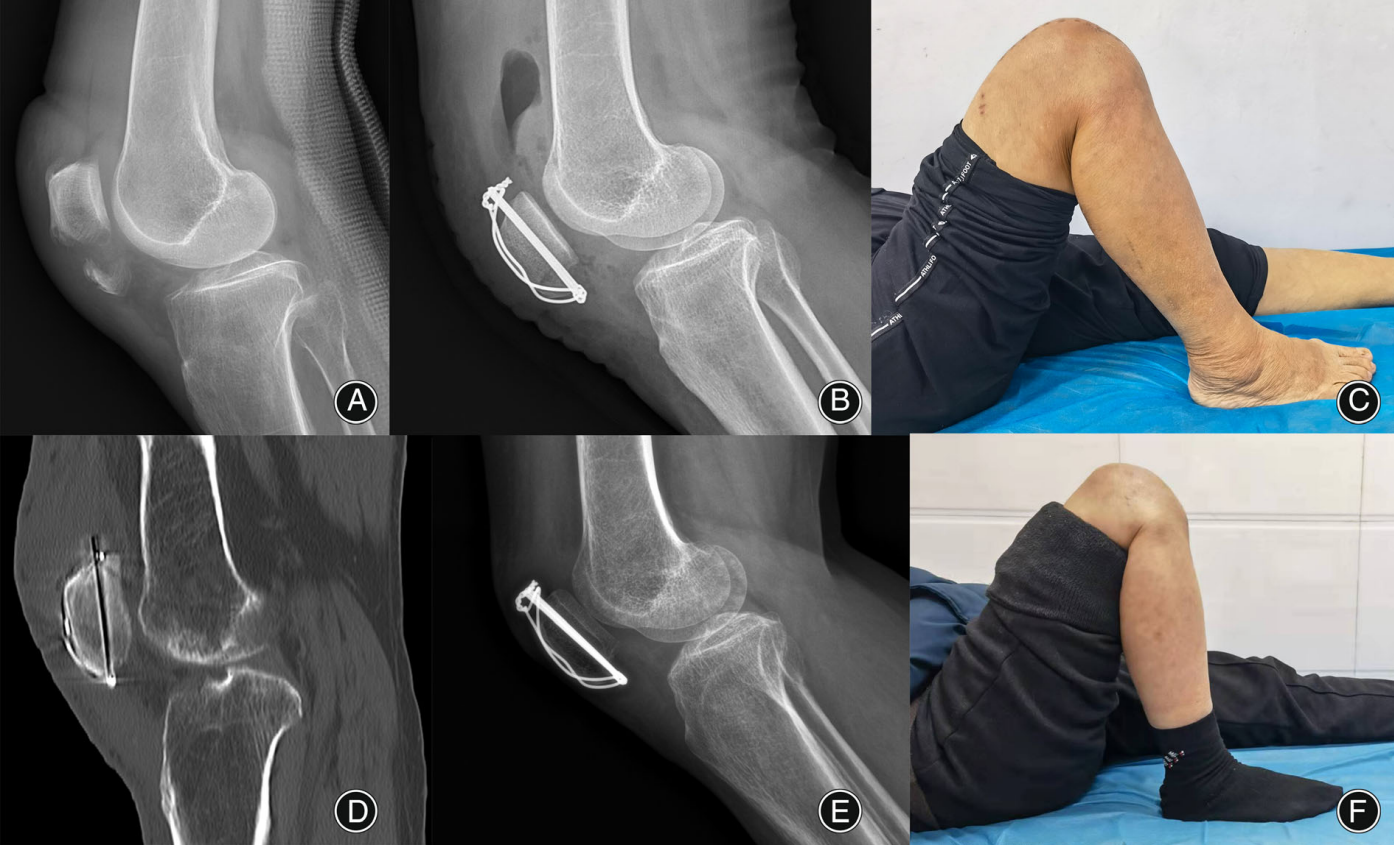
A 53‐year‐old male patient was diagnosed with an inferior patellar pole fracture on radiography (A). Postoperative lateral radiograph of the knee. The bone pin was not inserted through the inferior pole of the patella but below it into the proximal fracture fragment (B). Knee flexion over 90° at 2 weeks postoperatively (C). CT at 9 weeks postoperatively showing complete disappearance of the fracture line with bone union.
